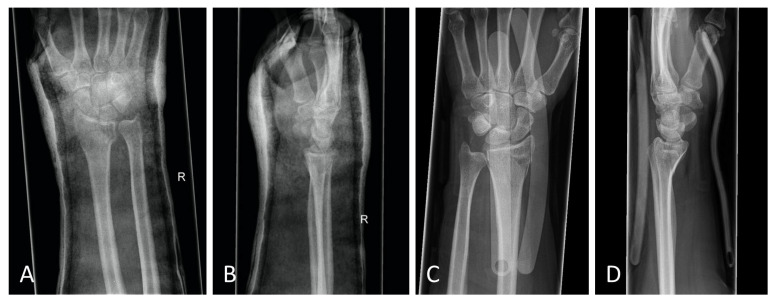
(A) Posteroanterior radiograph with cast. (B) Mediolateral radiograph with cast. (C) Posteroanterior radiograph with orthosis. (D) Mediolateral radiograph with orthosis. All radiographs were taken after reduction before surgery.
